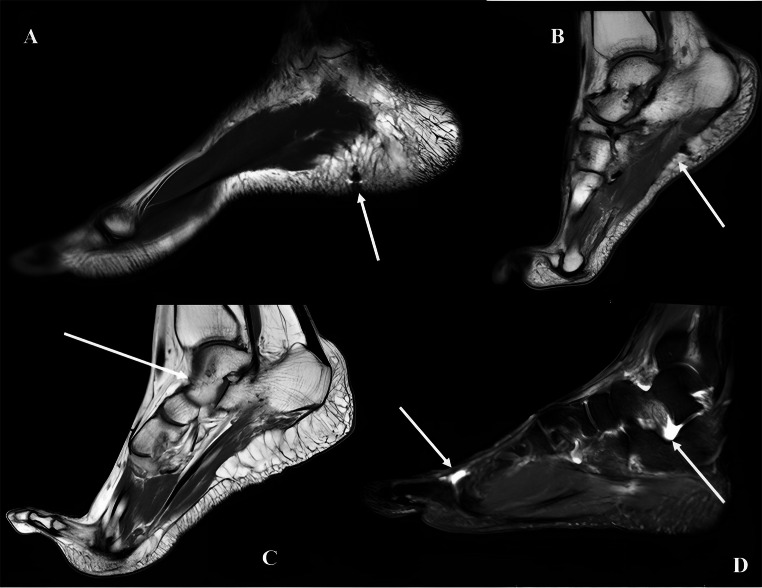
MRI of the foot findings of four subjects with clinical signs and history suggestive for previous falaka. (A) Sagittal TSE T2-weighted image of the right foot: thickening of the fatty tissue of the heel with irregular distribution of the fatty fibrous septa (thinned or absent) with an hypointense image in the subcutaneous area of the heel responsible for magnetic susceptibility artifacts who refers likely to a foreign body (white arrow). (B) Sagittal TSE T1-weighted image of the right foot: thinning of the plantar fascia; slight thickening of the fatty tissue of the heel with irregular distribution of the fatty fibrous septa (thinned or absent) with an hypointense area who refers likely to fibrosis (white arrow); (C) Sagittal T1-weighted image of the right foot: severe thinning of the plantar fascia; significant thickening of the fatty tissue of the heel with irregular distribution of the fatty fibrous septa (thinned or absent); irregularity of the dorsal cortex of the talus bone (white arrow). (D) Sagittal STIR image of the right foot: thin fluid collect on the dorsal side of the 1 st metatarsophalangeal joint and under the Talus bone (white arrows)
